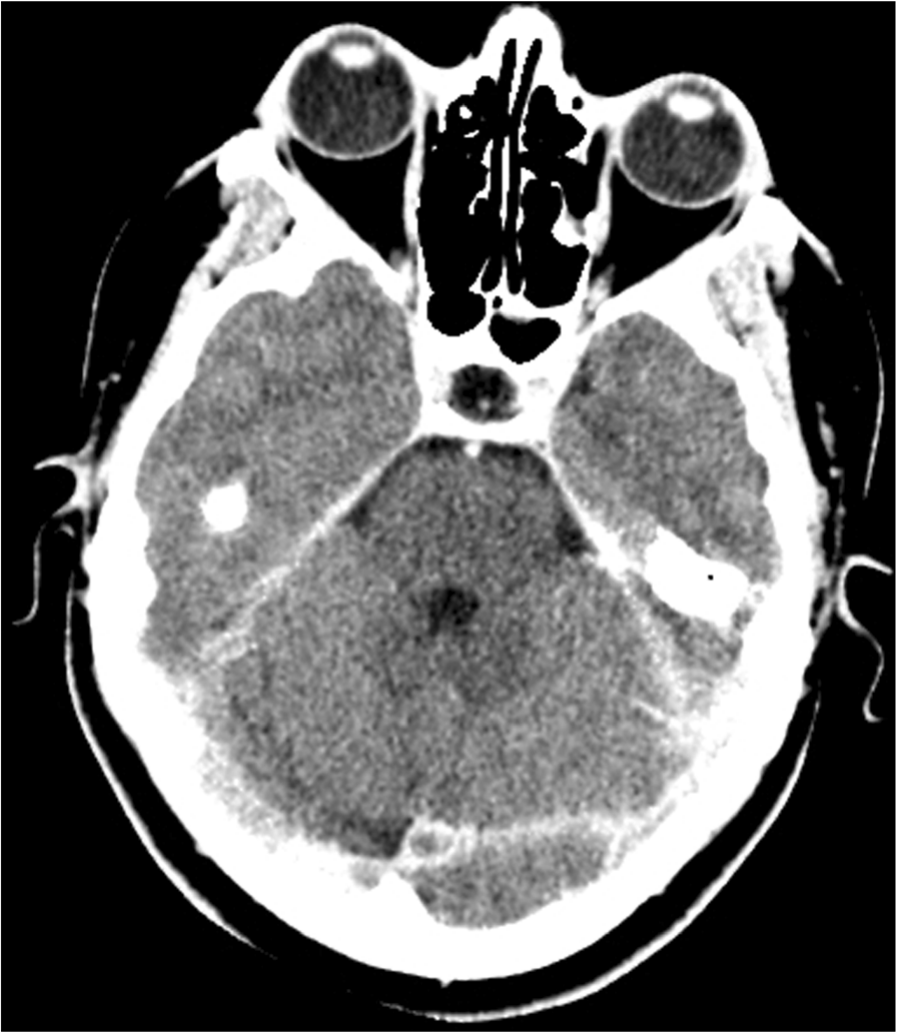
The cord sign shown in the computed tomography scan without contrast
